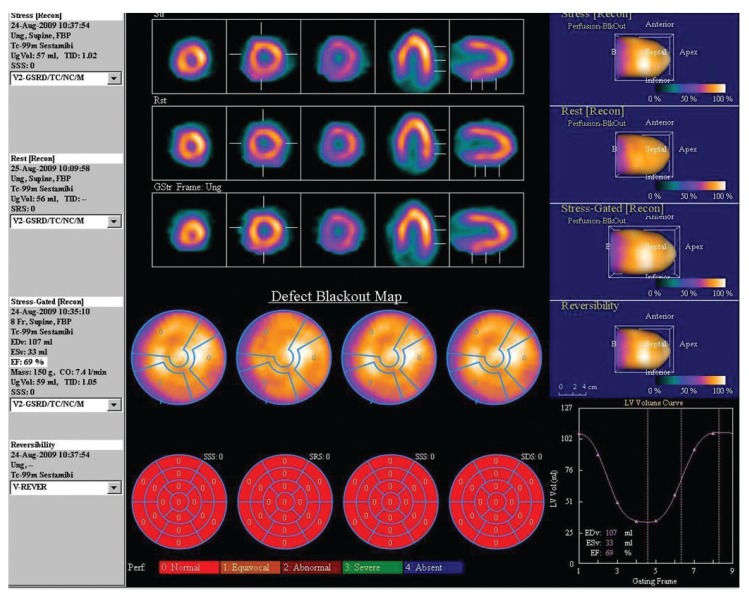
Myocardial perfusion scintigraphy and ‘bullseye’ plots of a 48-year-old male with diabetes, hypertension, dyslipidaemia and significant smoking history. His plasma homocysteine level was normal (7 μmol/l). There was normal perfusion at stress and rest, and the left ventricular ejection fraction was normal (69%).
